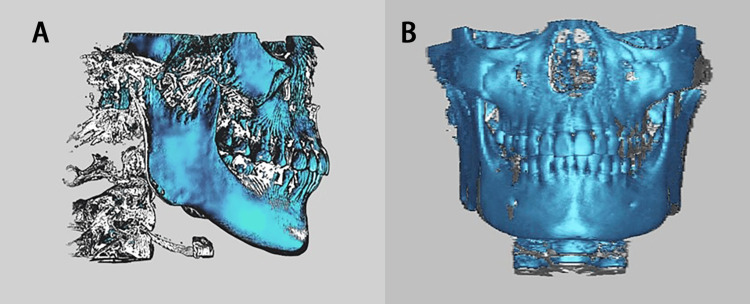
Landmark cone-beam computed tomography (CBCT) superimposition profiles The same four landmarks were included: ANS (the tip of the anterior nasal spine), PNS (the tip of the posterior nasal spine), OrL (the most inferior point of the left bony orbit), and OrR (the most inferior point of the right bony orbit). (A) Image overlap in the sagittal plane (B) Image overlap in the coronal plane
